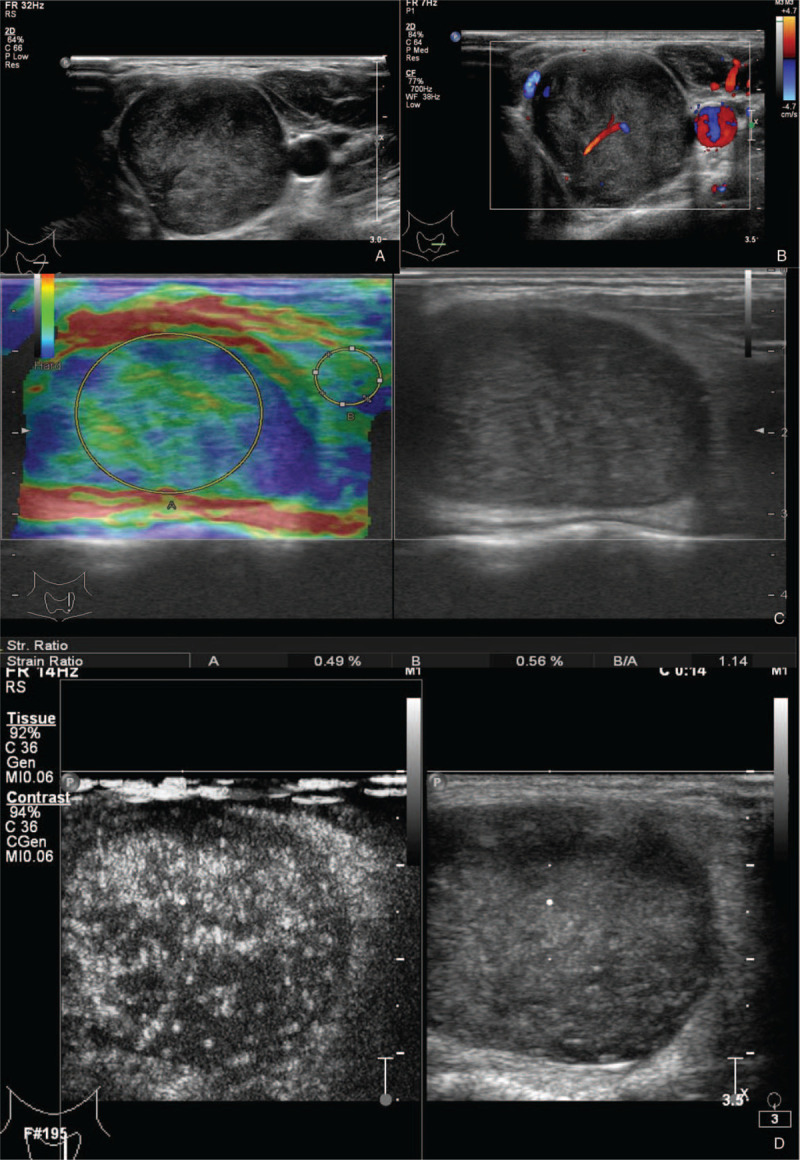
Multimodal ultrasound (US) imaging performed in a patient with a thyroid nodule suspicious for malignancy. The nodule size was 3.3 cm × 2.7 cm × 2.6 cm. (A) B-mode US revealed that the nodule was heterogeneous, hypo/iso-echoic with a sharp margin. (B) Color doppler showed intramural blood flow within the nodule with Adler grade I. (C) Ultrasonic elastography indicated that the lesion was medium-hard with a strain ratio of 1.14. (D) Contrast-enhanced ultrasound (CEUS) showed non-homogeneous enhancement (left) with “target sign” at baseline (right). B-mode and Color doppler were performed using the Philips IU 22 with a flat base 5 to 12 MHz probe and CEUS with a flat base 3 to 9 MHz probe. Ultrasonic elastography was performed using a HITACHI Vision 900 system.
